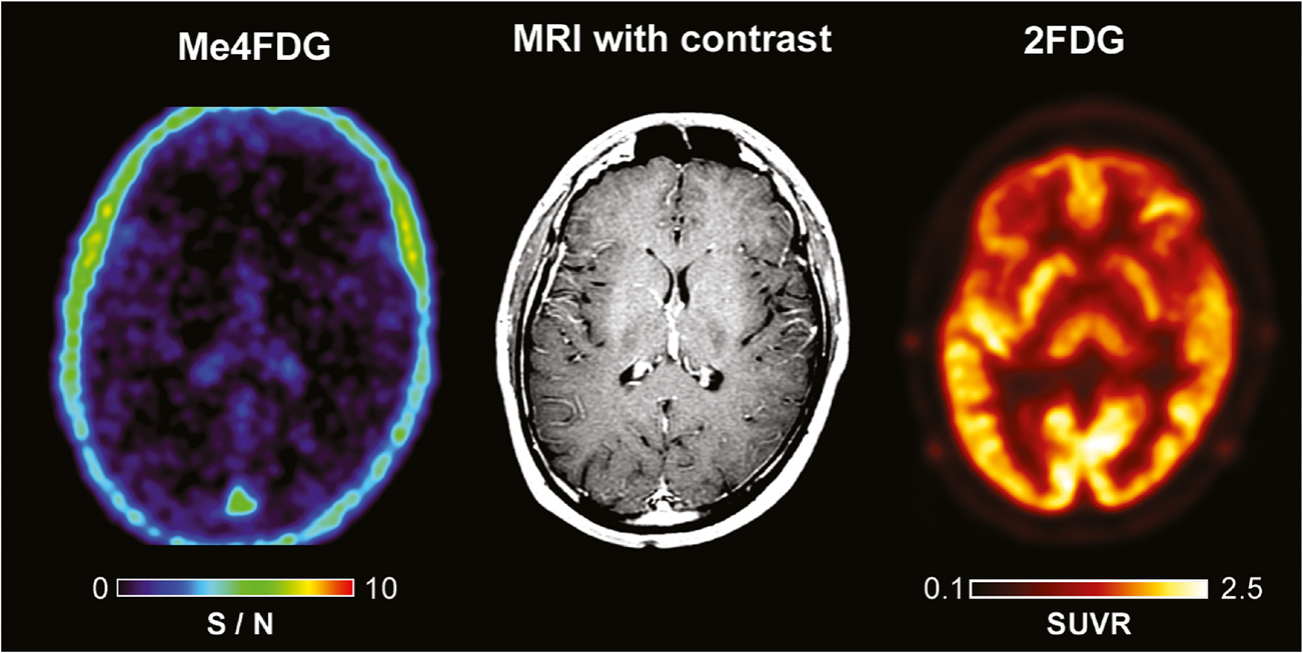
Me4FDG PET, MRI, and 2FDG PET scans on a WHO grade IV astrocytoma patient. The 57-year-old male patient has a 46-mm posterior corpus callosum astrocytoma. The Me-4FDG scan was conducted as for that on the control subject (Fig. 4) and shown as the S/N (SUVR/BG) ratio relative to the torcula on the NIH color scale. The standard clinical 2FDG PET brain scan is s for the “hot iron” on the SUVR based on a reference region of the brain. Taken from Kepe et al. [10]
